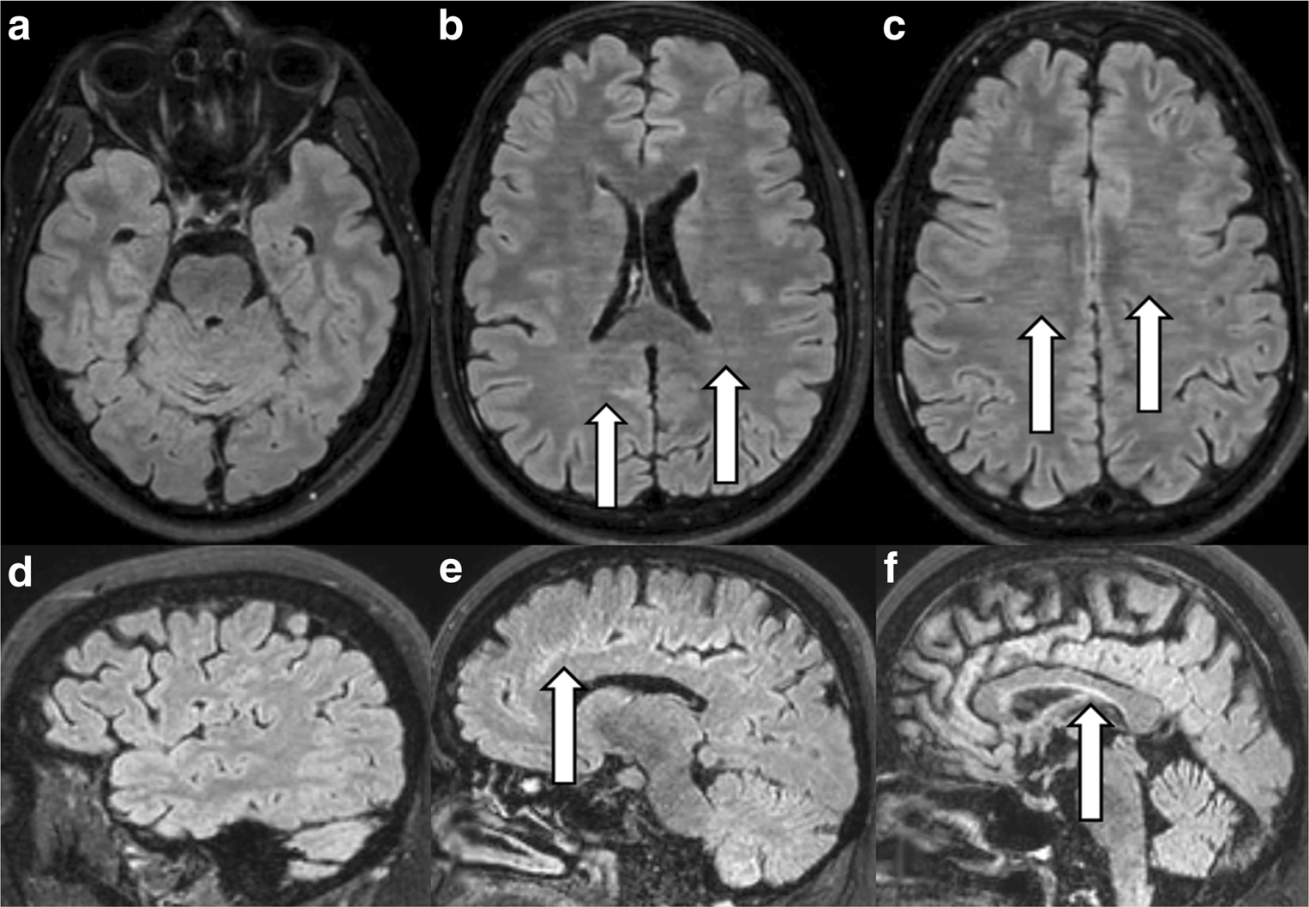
The streaky-linear artefact type B. Streaky-linear artefacts type B (*white arrows*) are horizontally oriented lines only in the centre of the transverse reconstructed image on 3D FLAIR (b, c) that are not visible or less visible along the image periphery (d) or infratentorially (a). There are faintly oblique lines on sagittal source images (e, f)
